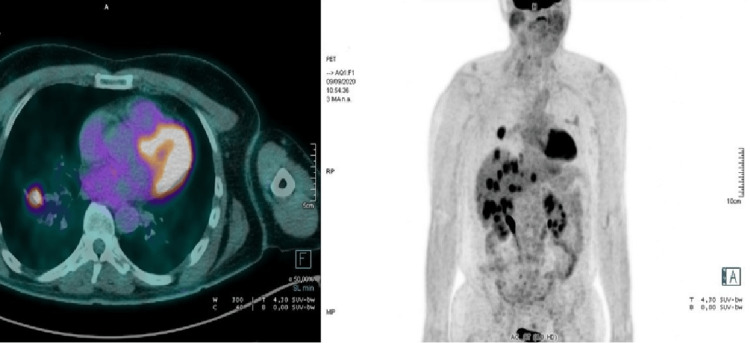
FDG-PET-CT image from September 2020. Right: full body FDG-PET-CT image from September 2020 showing lesions with FDG uptake in the lung and liver. Left: a close-up of the pulmonary lesion with high FDG uptake. FDG-PET-CT: fluorodeoxyglucose-positron emission tomography-computerized tomography
